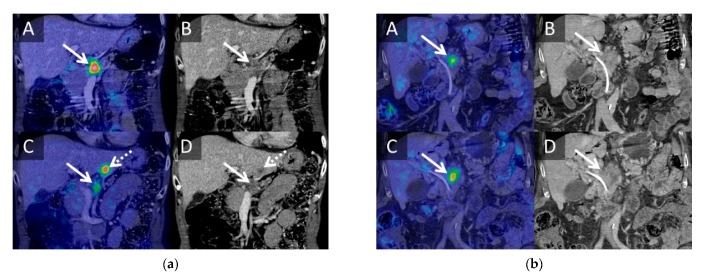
Representative ^18^FDG PET/CT images before and after IRE. (a) Sixty-one-year-old female with a T4 tumor in the head/body of the pancreas without nodal metastases. The patient was treated with three different chemotherapy regimens (FOLFIRINOX, XELOX, and gemcitabine) prior to inclusion (eight series in total). The patient was referred to IRE due to progression on chemotherapy. PET/CT images were performed before IRE (A,B) and 3 months after IRE (C,D). The patient had regression of SUVmax (9 -> 3.5 SUV_bw_), MTV (9 -> 1.86 cm^3^), TLG (37.51 -> 5.41 SUV_bw_cm^3^), and tumor size (23 -> 20 mm) at the primary tumor (solid arrow) but developed a PET-avid metastasis in liver segment III (dotted arrow). The patient received seven additional series of chemotherapy (XELOX and gemcitabine) after the 3-month scan and survived for 21 months after IRE ablation. (b) Seventy-seven-year-old male with a T4 tumor in the head/body of the pancreas with local nodal metastases. The patient was treated with six series of GEMCAP chemotherapy prior to inclusion. The patient was referred to IRE treatment due to progression on chemotherapy. PET/CT images were performed prior to IRE (A,B) and 3 months after IRE (C,D). The patient had increased SUVmax (5.27 -> 6.04 SUV_bw_), MTV (5.09 -> 10.66 cm^3^), TLG (16.31 -> 34.73 SUV_bw_ cm^3^), and tumor size (25 -> 33 mm) of the primary tumor (arrow) after IRE ablation. The patient did not receive any chemotherapy after IRE ablation, because of intolerance. The patient survived for 10 months after IRE ablation.
